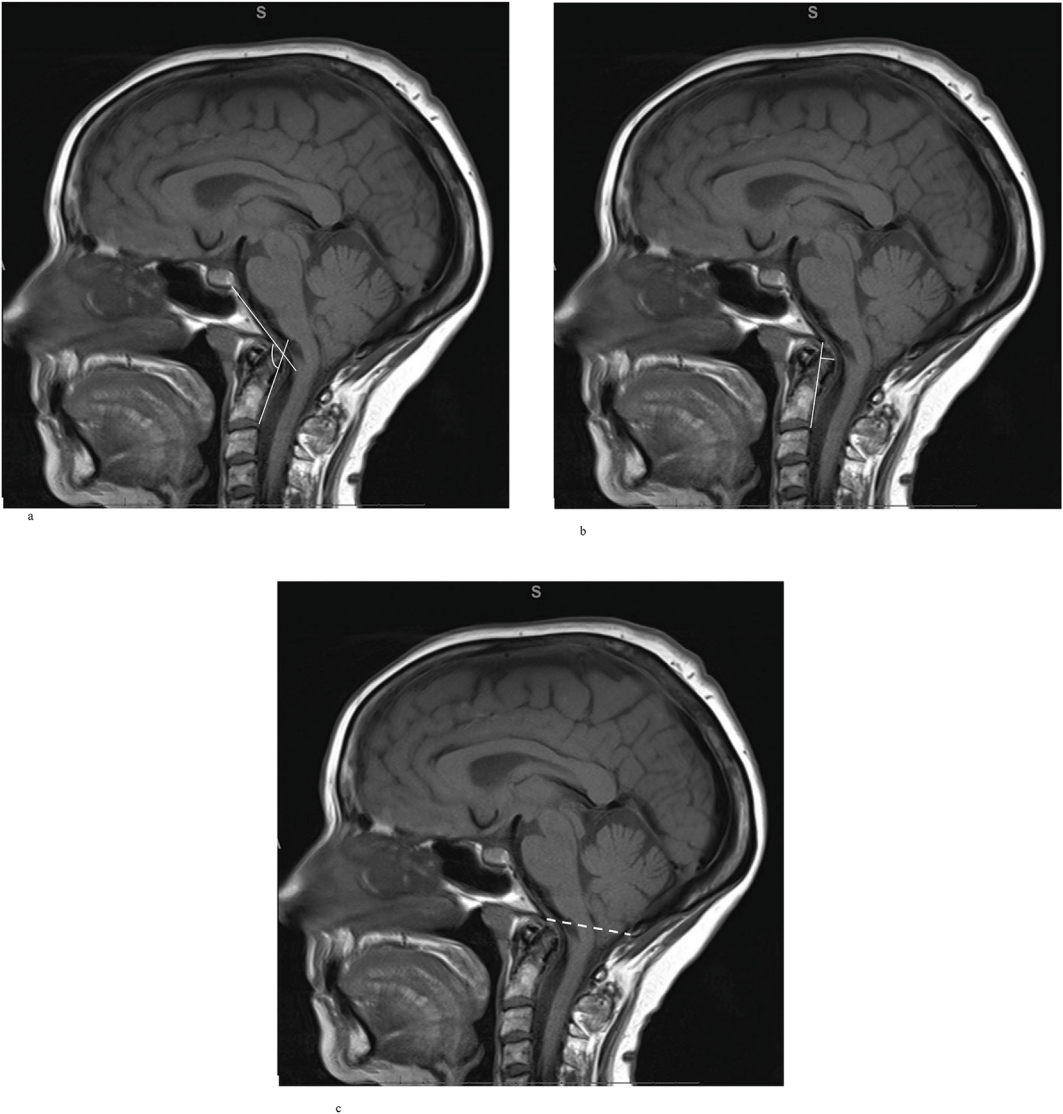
(a) The Clivoaxial angle (CXA) measures flexion at the craniocervical junction by measuring the angle between a line drawn along the clivus with that along the posterior margin of the odontoid peg and is said to be abnormal if on a flexion C-spine MRI it is < 135°. (b) The pBC2 line examines the dorsal protrusion of the odontoid peg, and is said to be abnormal if ≥ 9 mm. (c) McRae's line is the line drawn across the inferior margin of the foramen magnum, and is used to measure tonsilar descent.
